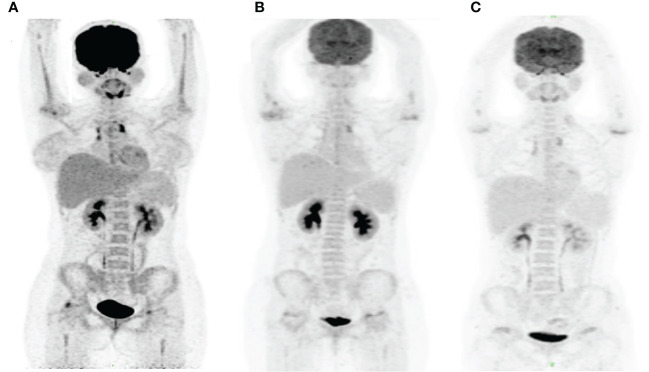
PET/CT images in this patient. The PET/CT images were obtained at month 3 before HSCT (A), month 2 after HSCT (B) and month 13.5 after HSCT (C). Panel (A) shows the increased tracer uptake in anterior superior mediastinum and new lesions of hypermetabolic focus in the bilateral femoral head and right humeral head. Panels (B, C) demonstrate a persistent complete remission after HSCT.
